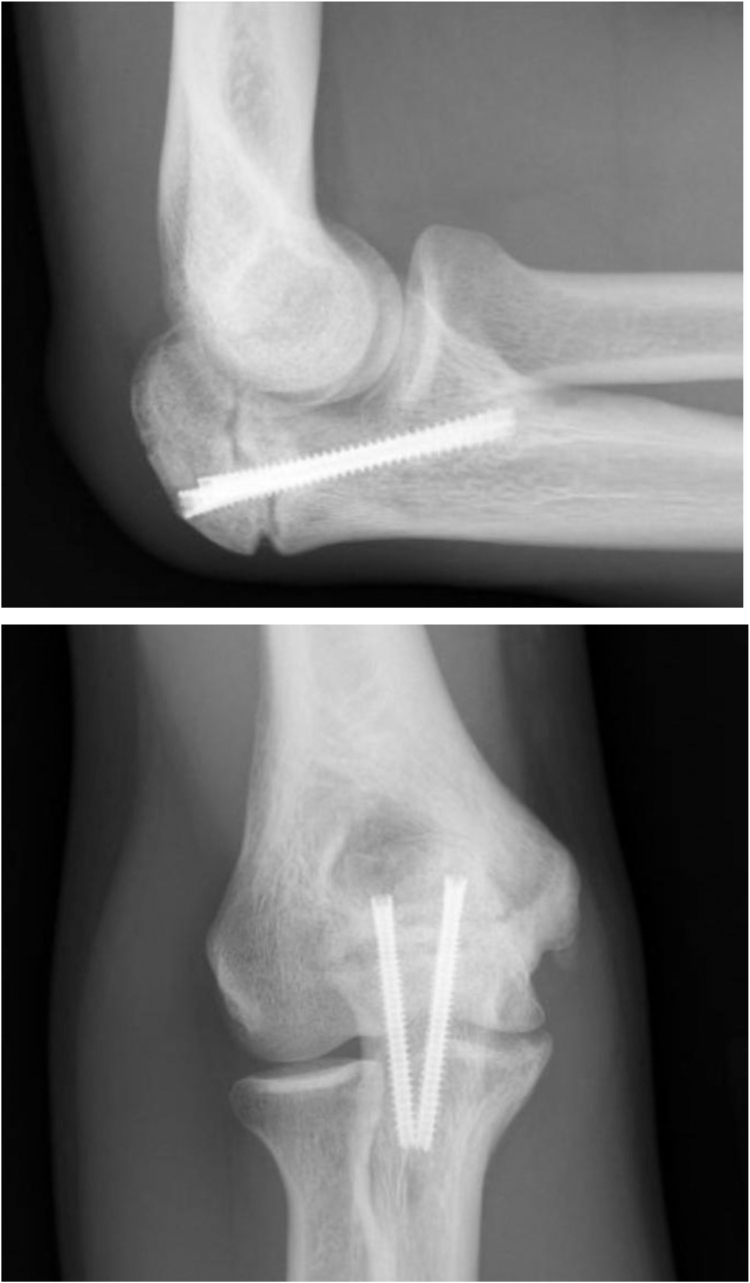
3-month postoperative plain films: well-maintained alignment of hardware with minimal callus formation and persistent nonunion of fracture edges.
